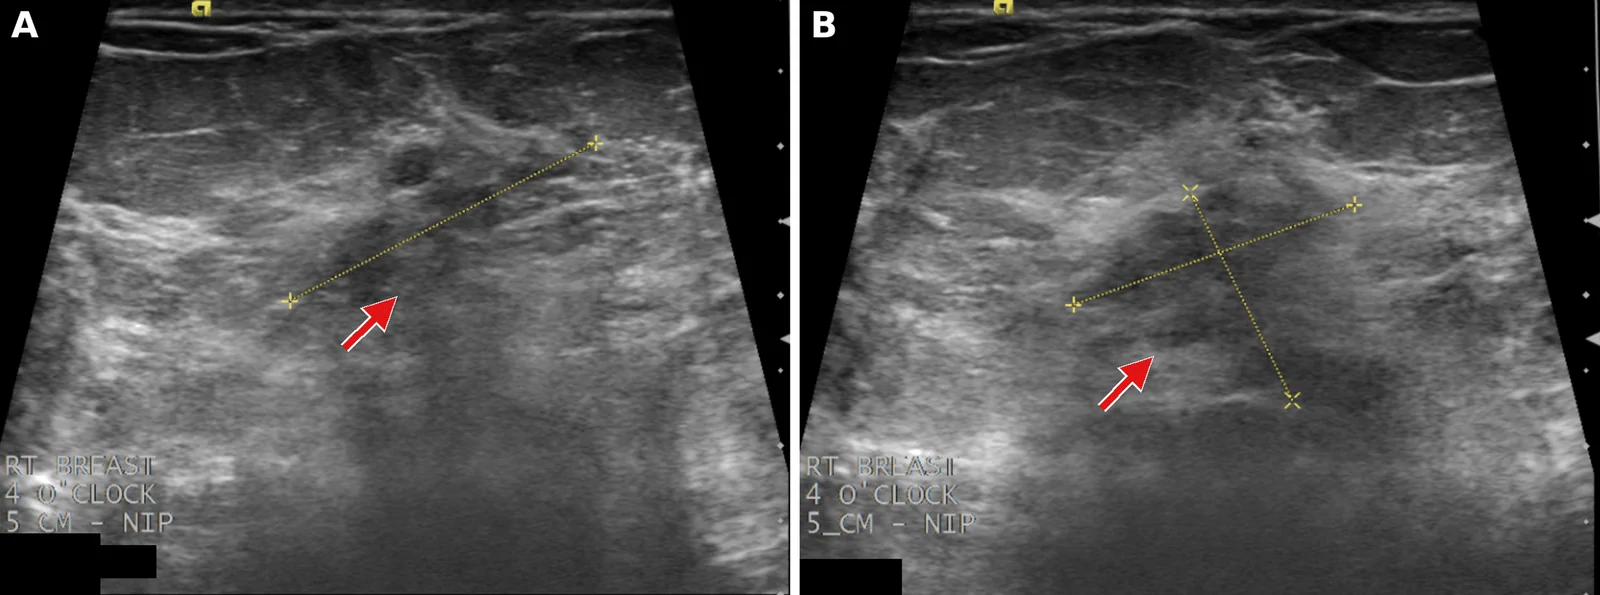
Targeted ultrasound of the right breast at the 4 o'clock position. Grayscale images in two orthogonal planes (A, B) at the 4 o'clock position, 5 cm from the nipple, demonstrate an irregular hypoechoic mass (red arrows) measuring 23x20x15 mm between the calipers, corresponding to the mammographic mass.
